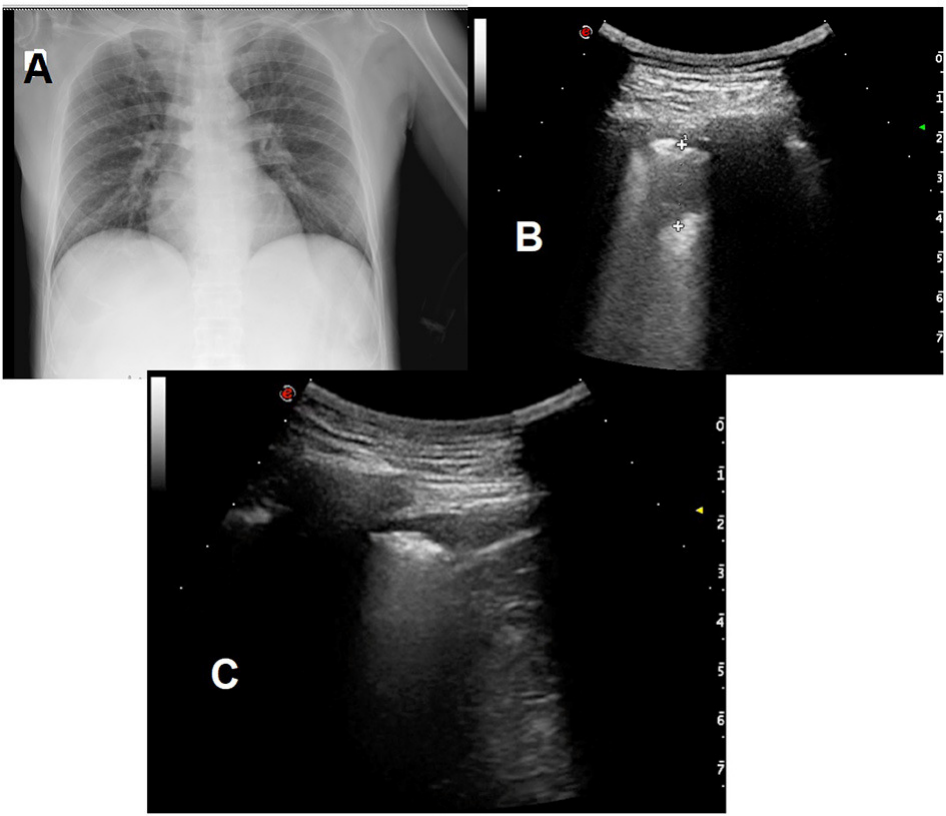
(A) Antero-posterior bedside X-ray showing no pulmonary consolidation and no apparent pleural effusion. (B) Posterior left basal LUS scan showing a small subpleural thickening of 1.5 cm. (C) hypoechoic consensual homolateral pleural effusion (depth: 9 mm) in the diaphragmatic costophrenic sinus.
